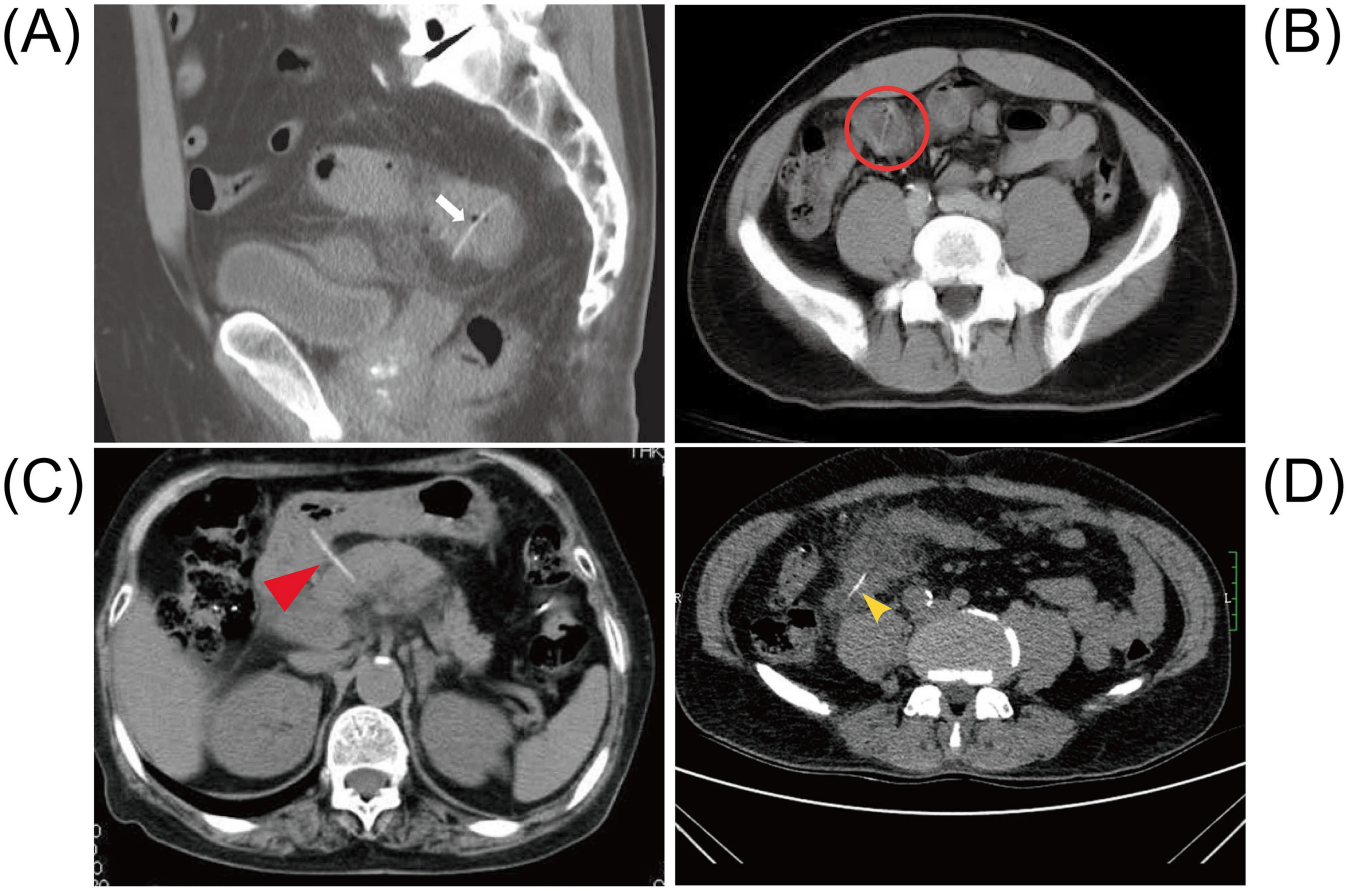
Abdominal CT after intestinal perforation caused by a fishbone. (A) The abdominal CT shows a radiopaque linear foreign body penetrating through the ileocecal wall (white arrow). Adapted from reference (108) with permission from J-STAGE. (B) The non-contrast abdominal CT shows a 26-mm long radiolucent linear shadow located within thickened bowel walls at both ends in the distal ileum (red circle). Adapted from reference (23) with permission from Baishideng. (C) The CT scan reveals a linear calcified body that appears to penetrate the posterior wall of the duodenal bulb and extends into the head of the pancreas. Adapted from reference (140) with permission from Springer Nature. (D) The abdominal CT shows patchy exudation and high-density shadow stripes in the right lower abdomen (yellow arrow). Adapted from reference (141) with permission from Springer Nature.
